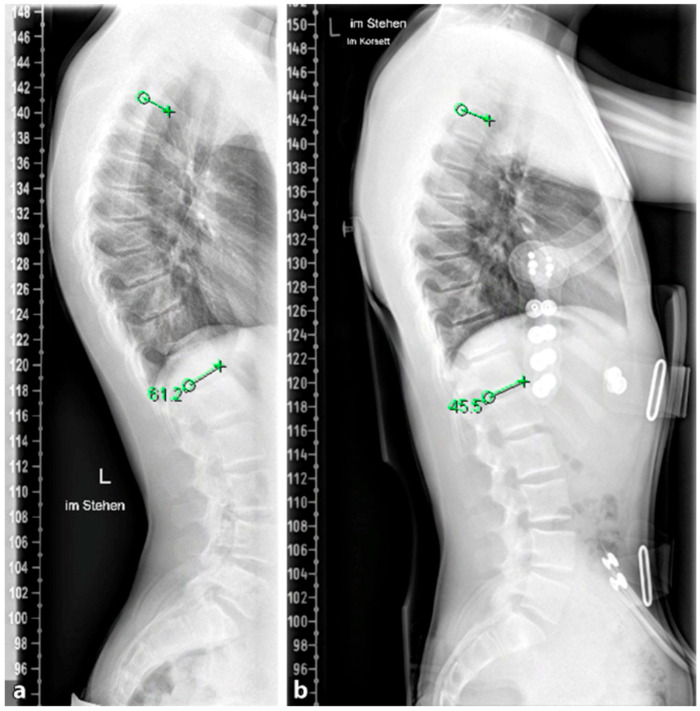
(a) X-ray of the full spine lateral view without brace, (b) Correction of mild hyperkyphosis in reclination brace (from 61.2° to 45.5°) (reprinted with permission [23]).
